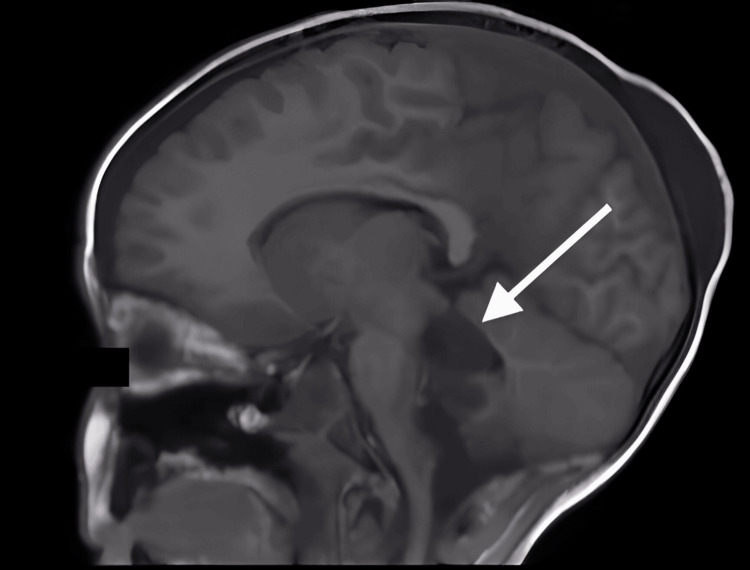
MRI image in the sagittal plane of the tumoral process in the posterior cerebral fossa
